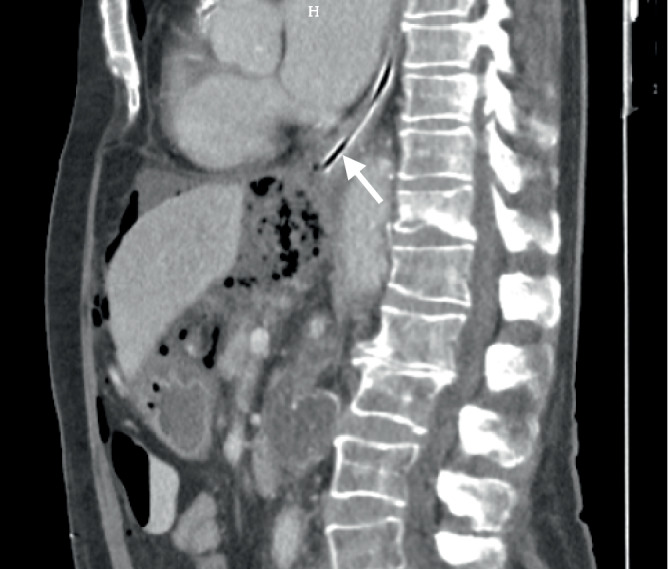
CT-Aufnahme in Sagittalebene: Es zeigen sich das beschriebene Luft-Flüssigkeits-Gemisch retrohepatisch sowie wenig freie Luft intraabdominell. Auf Zwerchfellebene scheint die NGT korrekt im Ösophagus zu liegen (*Pfeil*). (Abbildungsquelle: Aus dem klinischen Image- und Bilddokumentenfokus der Klinik für Radiologie und Nuklearmedizin; Otto-von-Guericke-Universität mit Universitätsklinikum, Magdeburg, Deutschland)
